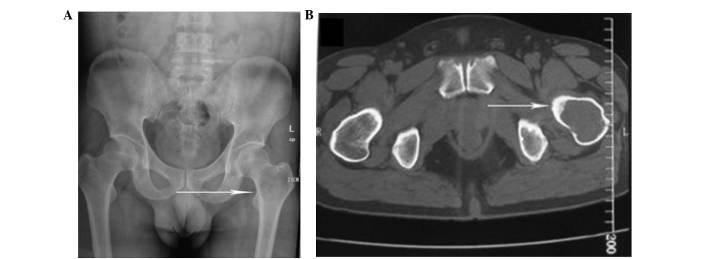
(A) Radiograph and (B) computed tomography (CT) images of the pelvis showing a low-signal intensity in the left femoral trochanter (white arrow).
